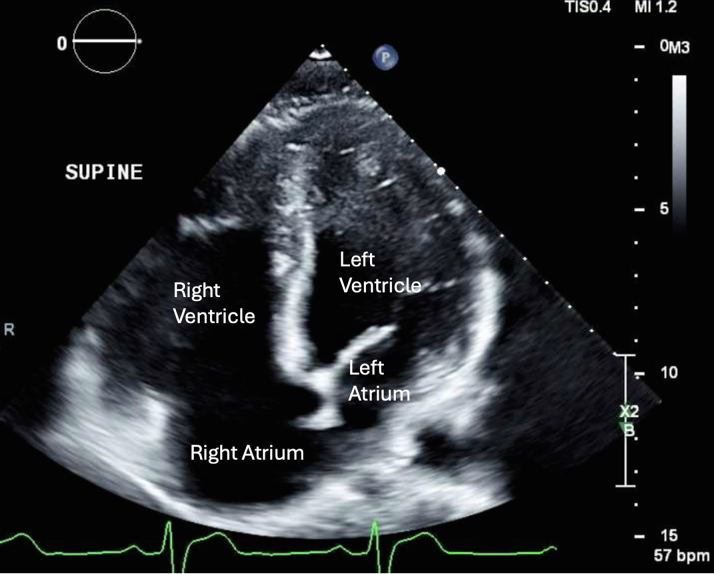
Apical 4-Chamber View Demonstrating Mild Right Ventricular Dilation
